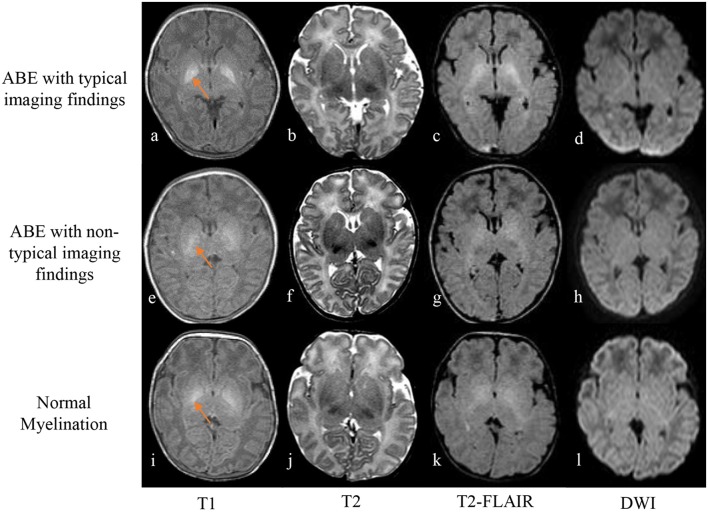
Axial T_1_-weighted spin echo image (a) shows strikingly hyperintense signal in the globus pallidus with well-defined boundary in a neonate of 10 days old with ABE (a–d), while T_2_-weighted fast spin echo image (b) and FLAIR image (c) show slightly and moderately high signal intensity, compared with the signal intensity of the surrounding basal ganglia region, respectively. No abnormal signal intensity was detected on DWI (d). However, in cases of a 4-day-old neonate with radiologically untypical ABE (e–h) and a case of 9-day-old ABE-alike neonate with normal myelination (i–l), imaging findings include hazy slight hyperintensity on T_1_-weighted image (e,i), iso-intensity on T_2_-weighted image (f,j), slightly high signal intensity on T_2_-weighted FLAIR images (g,k), and no obvious abnormal signal intensity on DWI (h,l) in the region of globus pallidus with referencing the signal intensity of the surrounding region of basal ganglia.
